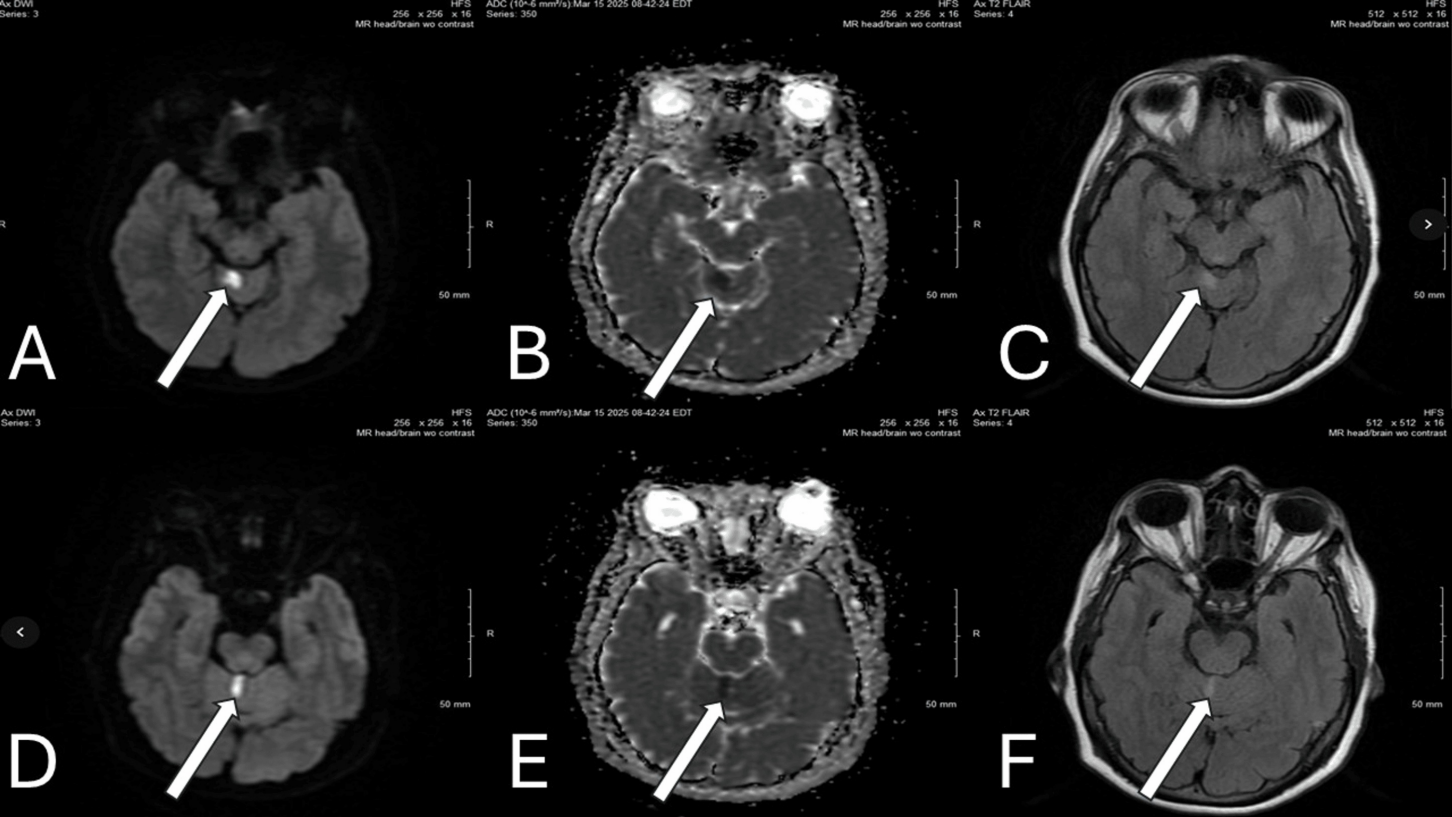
Brain MRI axial images (A and D: DWI, B and E: ADC, C and F: T2 FLAIR) showing small foci of diffusion restriction in the right superior vermis. Hyperintense signals are appreciated on the T2 FLAIR and DWI with hypointense signals on ADC, indicative of an acute infarct. Arrows are pointing to the acute infarcts. ADC: apparent diffusion coefficient, DWI: diffusion-weighted imaging, MRI: magnetic resonance imaging, T2 FLAIR: T2-weighted fluid-attenuated inversion recovery
